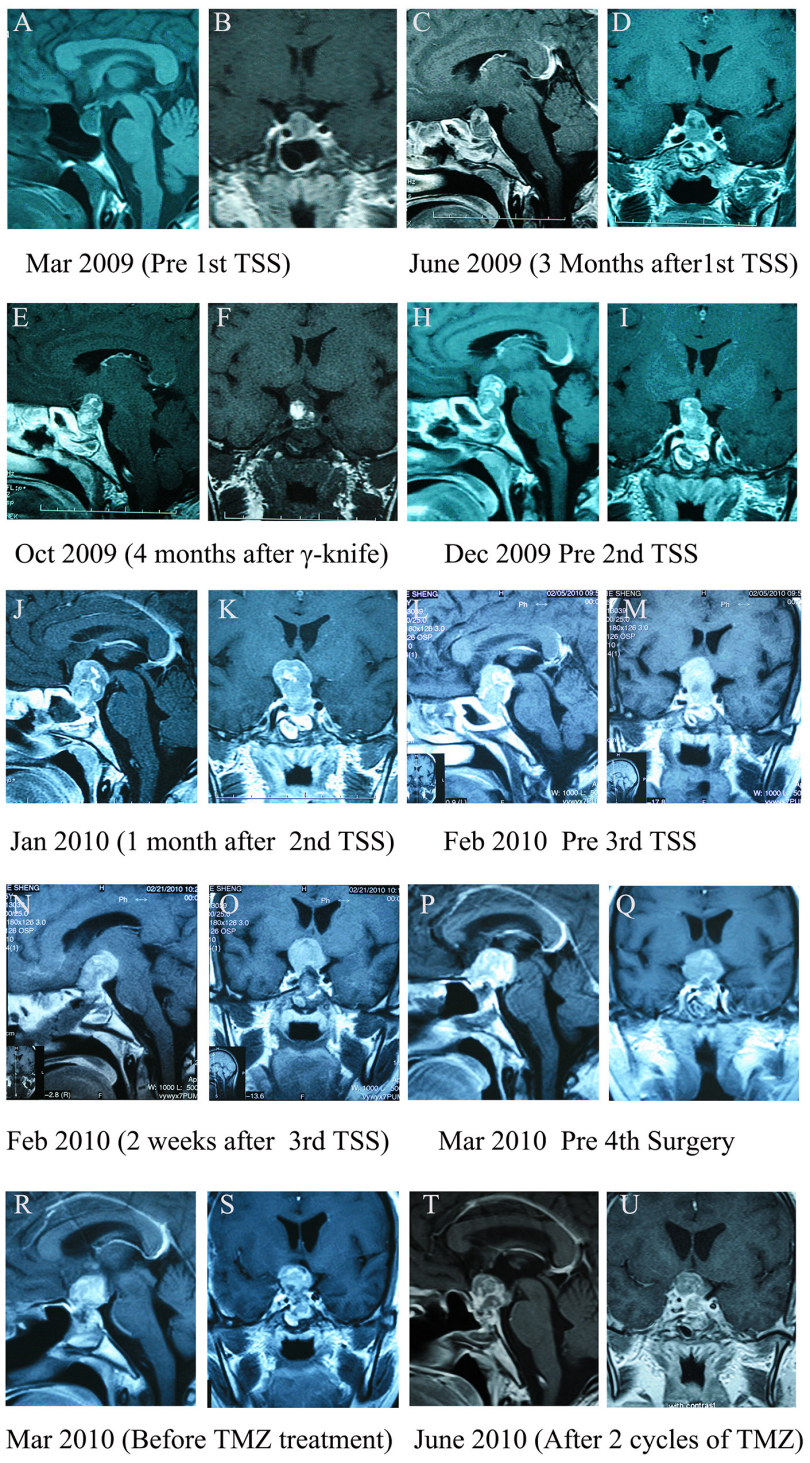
Pituitary MRI images for the case one patient A and B. Pre-operative T1 weighted images. C and D. 3 months after the first transsphenoidal surgery (TSS). E and F. 4 months after gamma knife surgery (GKS). H and I. 6 months after GKS. J and K. 1 month after the second TSS. L and M. 2 months after the second TSS. N and O. 2 weeks after the third surgery. P and Q. 1 month after the third surgery. R and S. 2 weeks after the fourth operation, before TMZ treatment. T and U. After two cycles of TMZ therapy.
